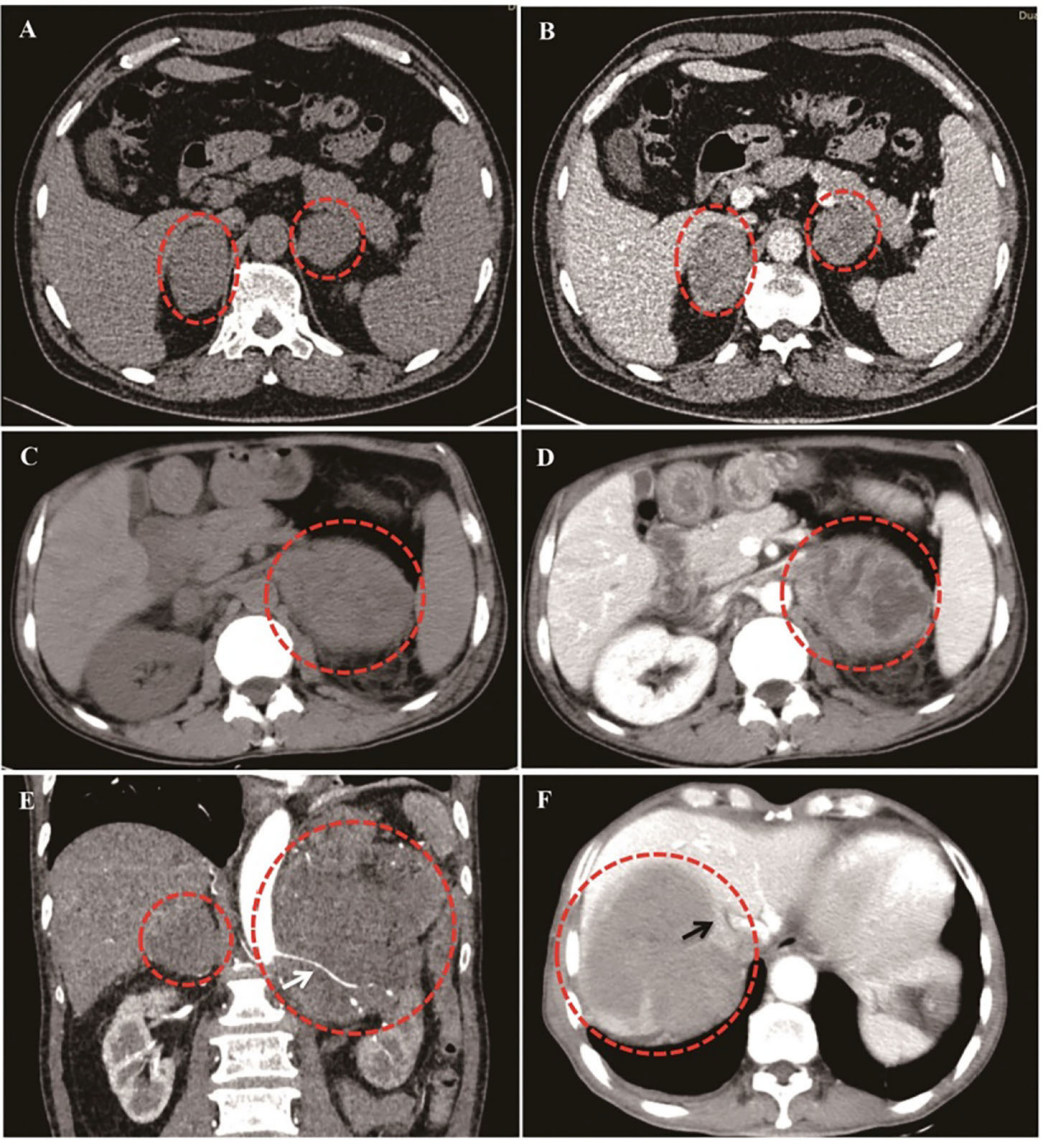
Different CT imaging features of PAL patients. (A,B): Bilateral PAL with oval/round shape, well-defined edge, moderately enhanced in parenchyma phase, and homogeneous enhancement patterns. (C,D): Bilateral PAL with liquidation/necrosis in the left tumor mass, which was obvious after contrast by iodamide. (E): Lymphoma cells surrounded the renal artery without invasion (White arrow). (F): Right lymphoma mass invaded inferior vena cava (Black arrow).
